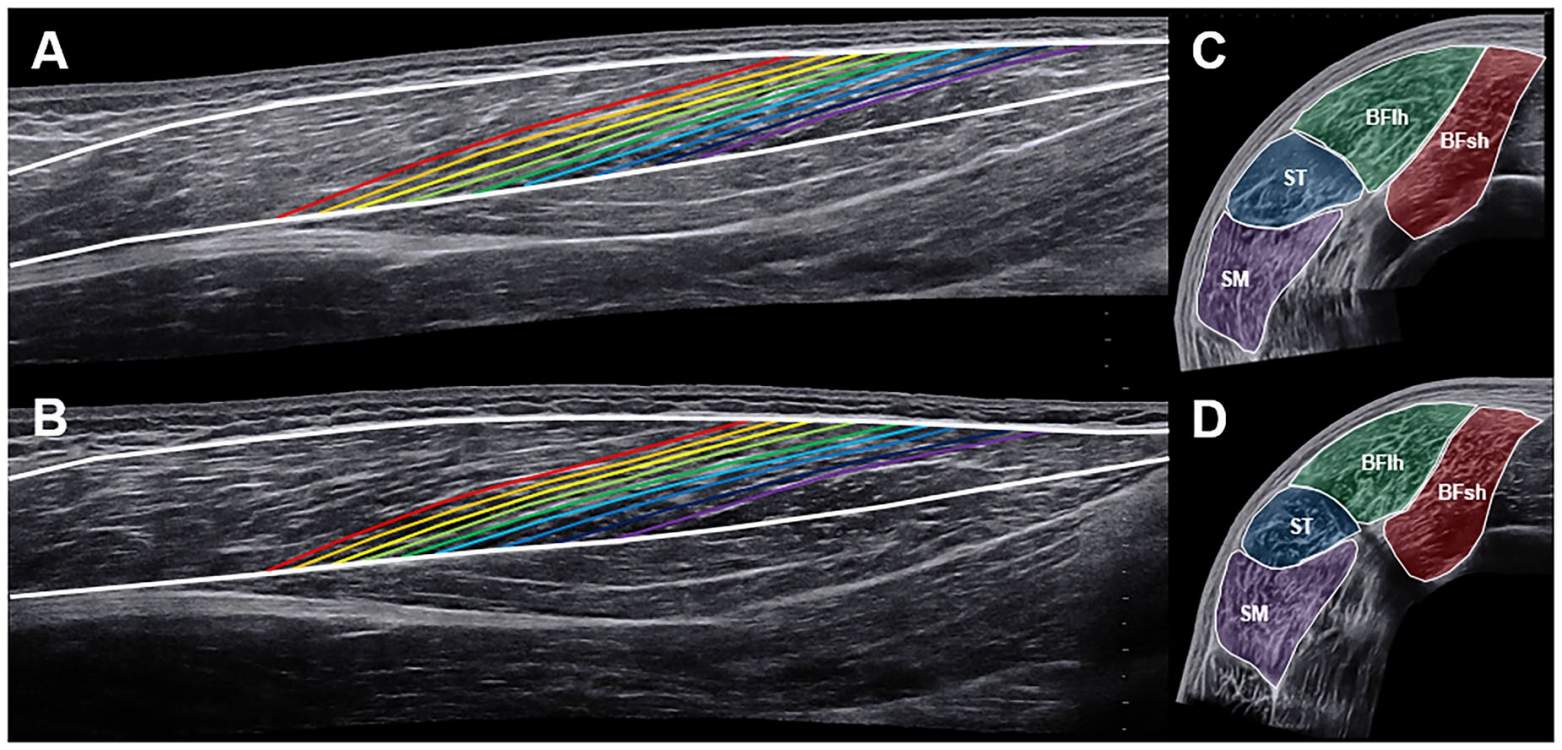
Representative ultrasound scans showing the biceps femoris long head muscle architecture at baseline (A), at the 4-year follow-up (B), as well as the hamstring anatomical cross-sectional areas at baseline (C) and at the 4-year follow-up (D). BFlh = biceps femoris long head, BFsh = biceps femoris short head, ST = semitendinosus, SM = semimembranosus.
